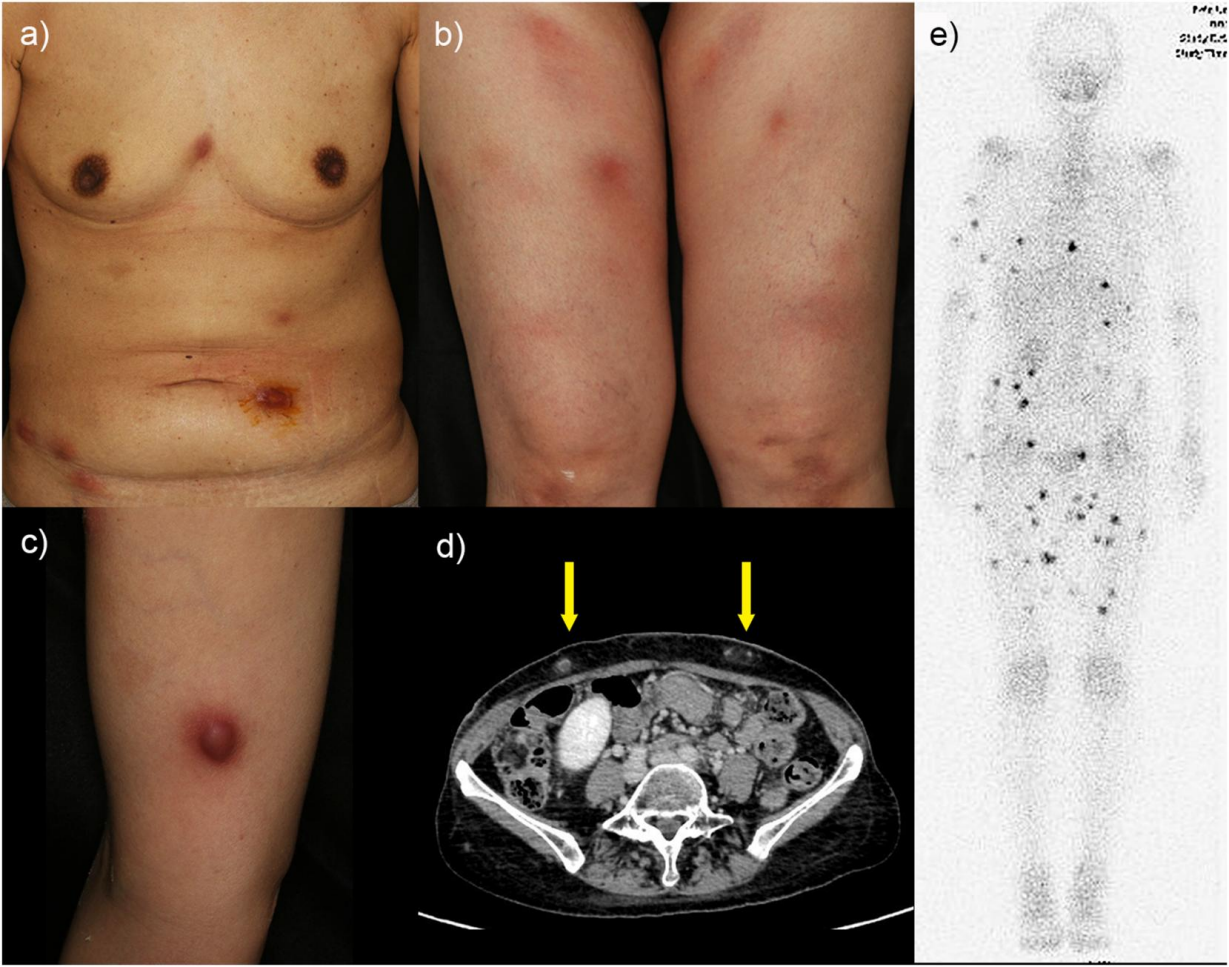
(a–c) Clinical images. Erythematous subcutaneous nodules are distributed on the whole body. The nodules were firm and tender. (d) The nodules (yellow arrow) were localised in the subcutaneous layer on computer tomographic scanning. (e) Gallium scintigraphy showed multiple nodular accumulations all over the body.
